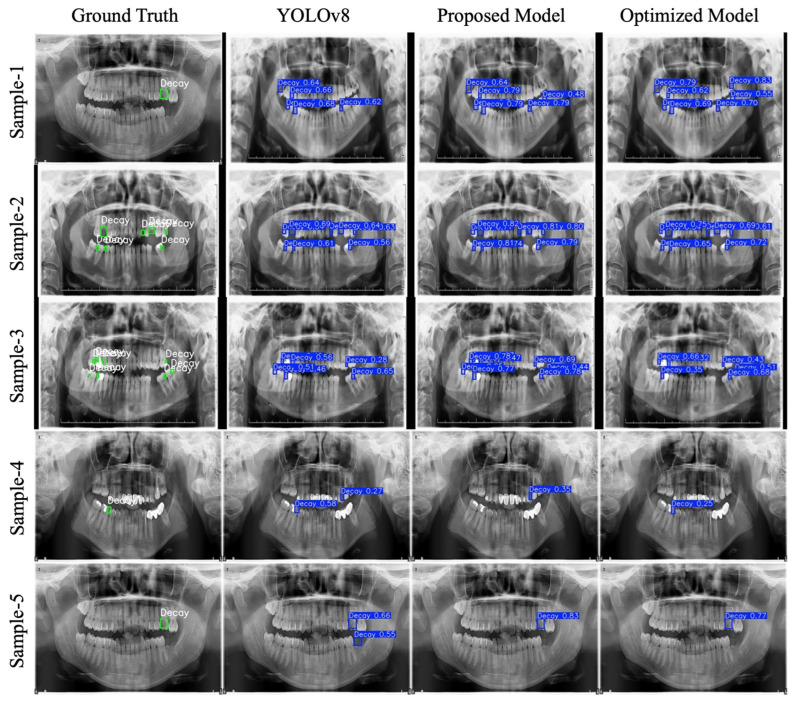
Comparative detection results for five representative panoramic radiographs. From left to right, the columns show expert ground-truth annotations (green bounding boxes), YOLOv8 predictions, predictions of the ConvNeXtV2-enhanced proposed model, and predictions of the GA-optimized model (blue bounding boxes with confidence scores).
